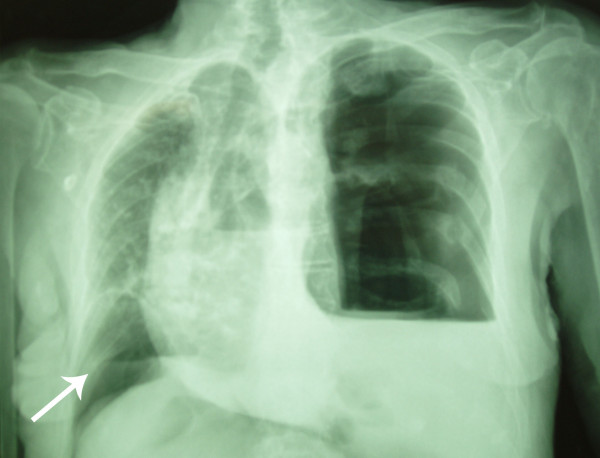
Initial chest x-ray showing a left tension pneumothorax with shift of the mediastinum to the right, pleural effusion left, basal dorsolateral rib fractures. There's also air visible under the right diaphragm (arrow).
